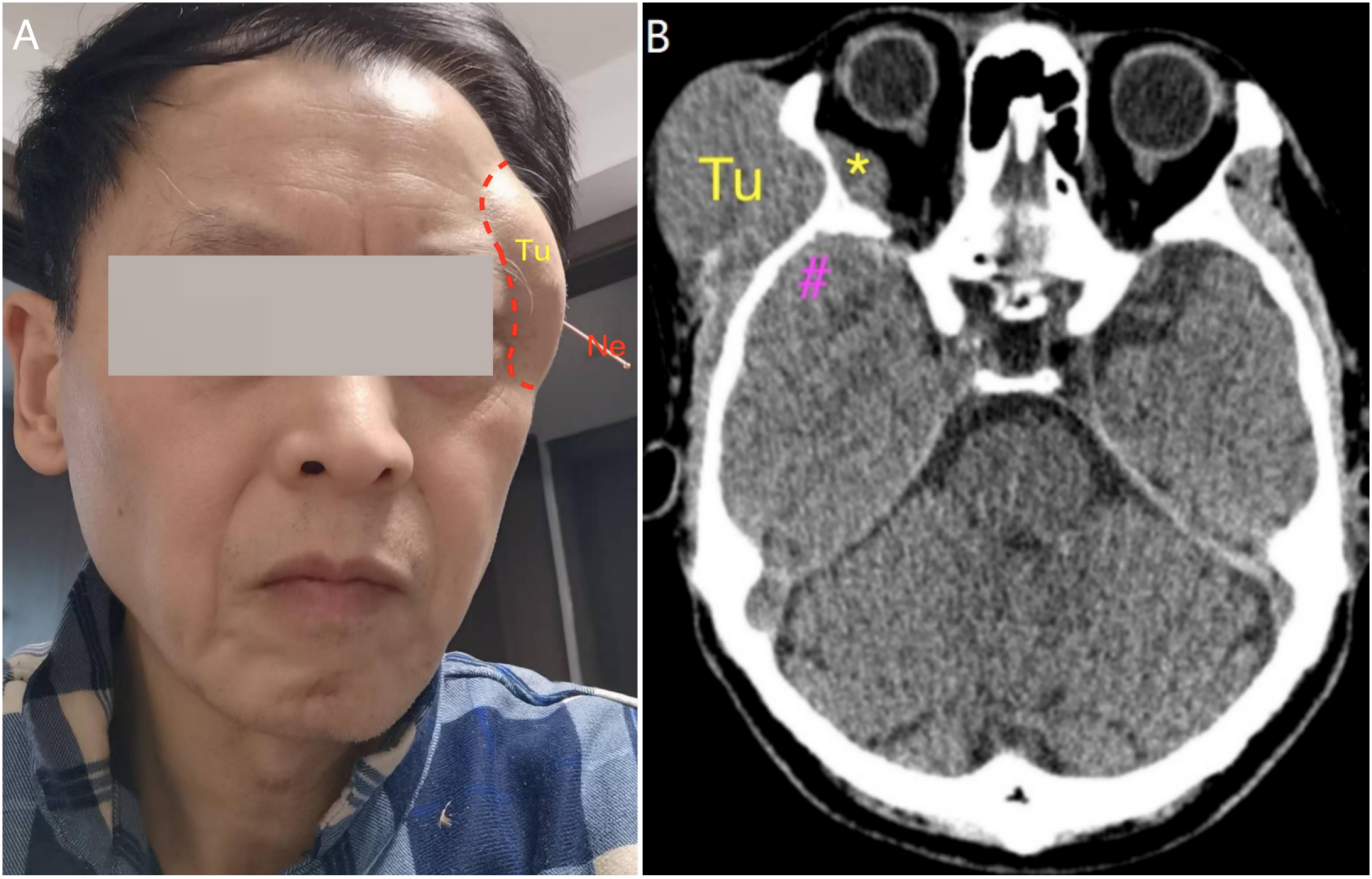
Clinical photograph and preoperative non-contrast CT. (A) Clinical photograph showing the right frontotemporal subcutaneous mass; the patient had previously undergone acupuncture. (B) Representative axial non-contrast head CT demonstrating lesions in the frontotemporal subcutaneous tissue (Tu), the orbit (*), and the floor of the middle cranial fossa adjacent to the temporal pole (#); retained acupuncture needles are visible (Ne). Tu, tumor; *, intraorbital lesion; #, middle cranial fossa lesion; Ne, retained acupuncture needles.
